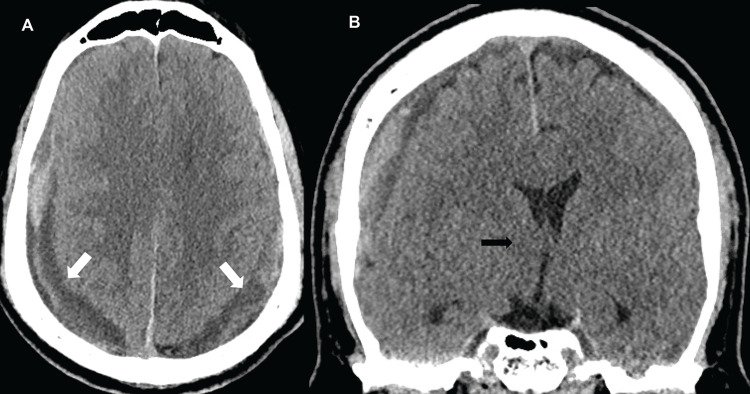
Emergency department presentation, non-contrast computed tomography of the head. (A) Axial plane. (B) Coronal plane. Enlarging bilateral subdural hematomas (white arrows) and right-to-left midline shift (black arrow).
